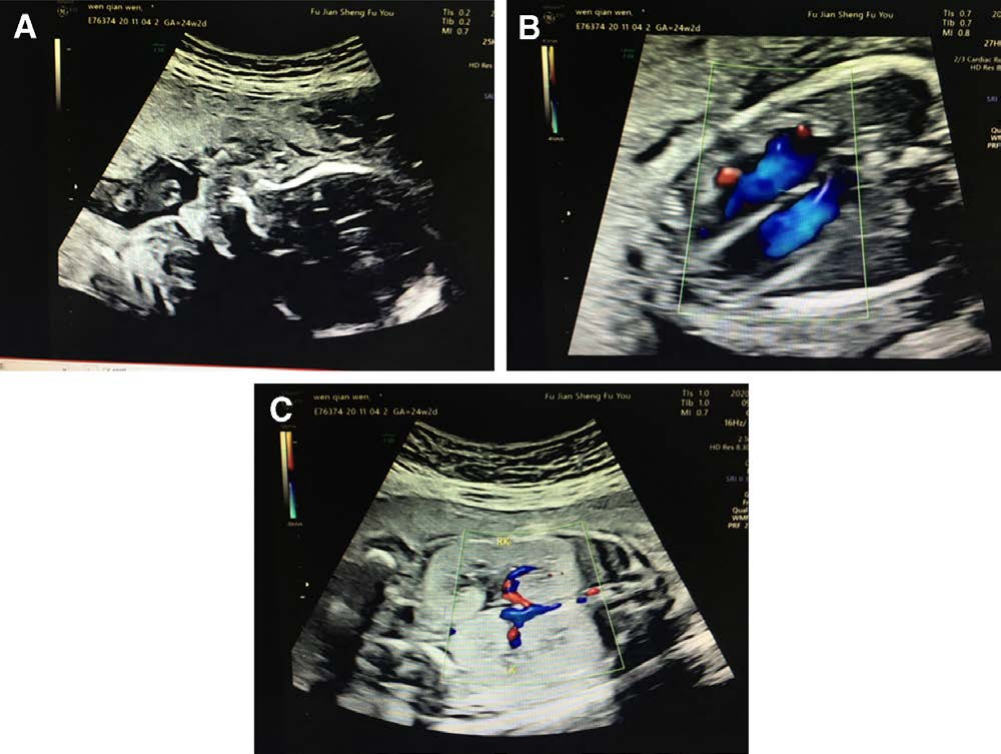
Intrauterine ultrasound phenotype of the fetus. (A) Ultrasound of the fetus at 24 ^+2^ gestational week; single pregnancy in utero. (B) Ultrasound of the fetus at 24 ^+2^ gestational week; both kidneys were enlarged, the echo was enhanced, and infantile polycystic kidney was suspected. (C) Ultrasound of the fetus at 24 ^+2^ gestational week; the amniotic fluid index was 2.9 cm, showing a slightly sparse state.
